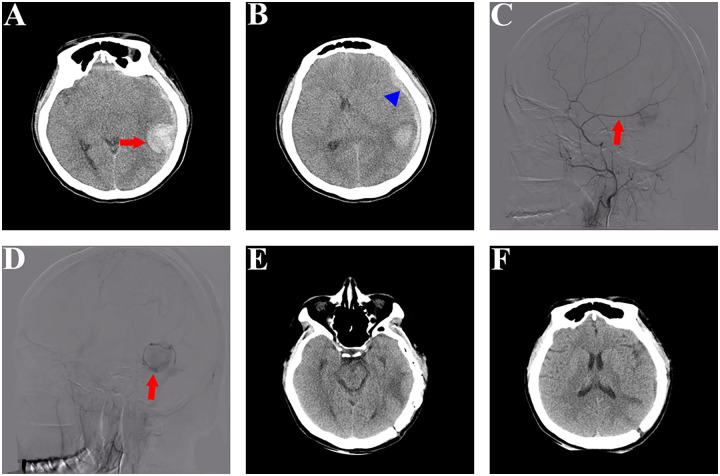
Preoperative CT, intraoperative DSA, and postoperative CT images. (A,B) Preoperative head CT showing left temporal lobe hemorrhage and left fronto-parieto-temporal acute subdural hematoma. (C,D) Intraoperative DSA demonstrated that the left temporal lesion was supplied by the temporal branch of the left middle meningeal artery, presenting a “ball-holding sign.” (E,F) Postoperative head CT confirming complete tumor resection and hematoma evacuation.
